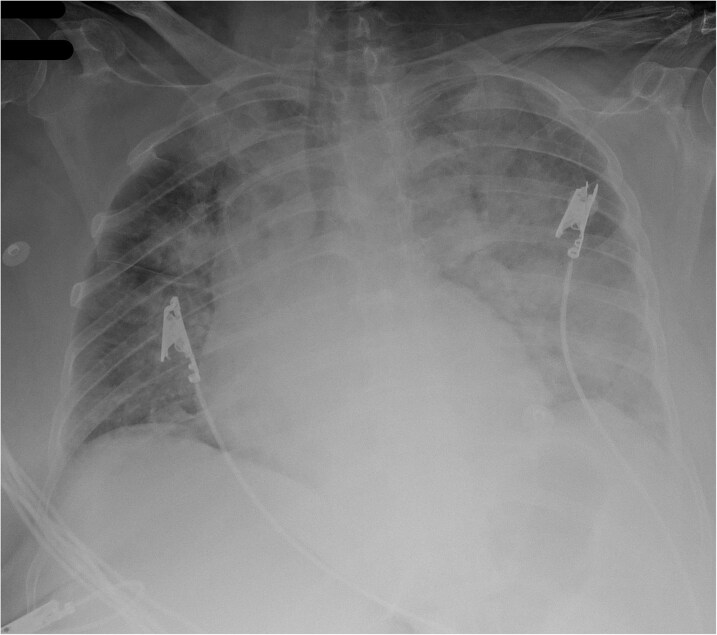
Admission chest X-ray revealed pulmonary congestion.
